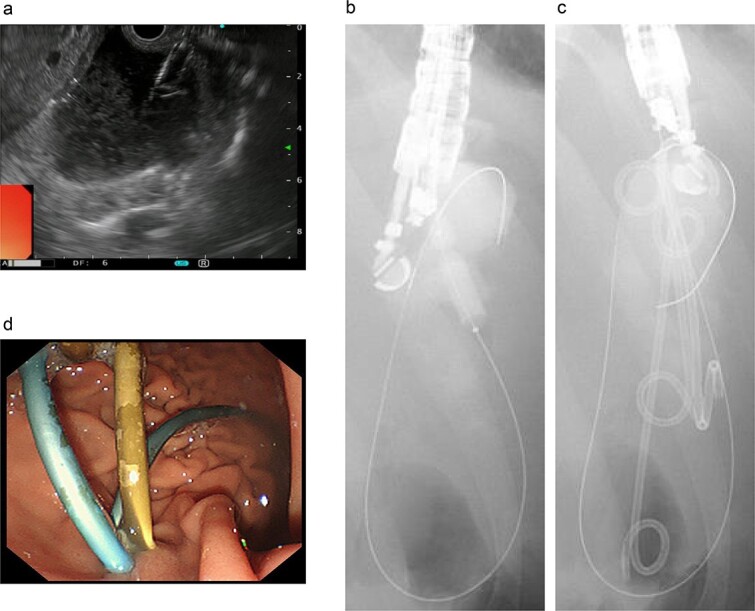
Endoscopic ultrasound-guided transgastric pseudocyst drainage. (a) Endoscopic ultrasound-guided puncture of the pseudocyst. (b) The posterior wall of the stomach was punctured, and the puncture hole was dilated with a balloon. (c) Placement of three tube stents through the puncture hole. (d) Placement of three tube stents of 7 Fr-7 cm and 7 Fr-4 cm × 2.
